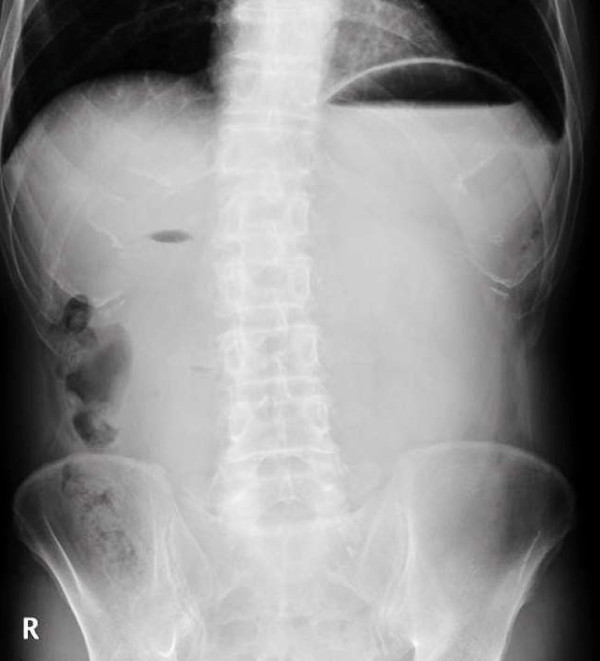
Abdominal X-ray showed distension sign of the stomach with air fluid level and duodenal gas.
